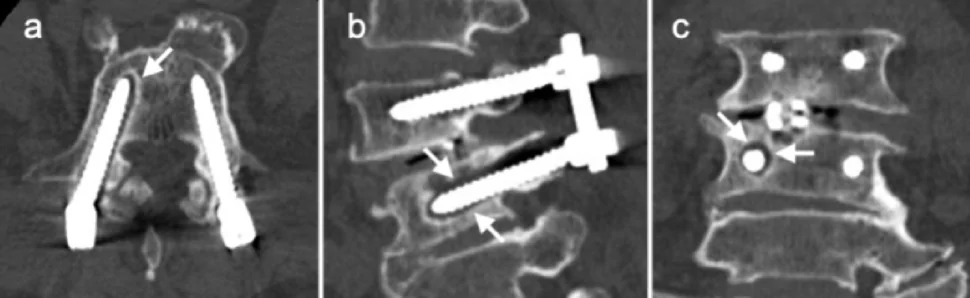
Assessment of pedicle screw (PS) loosening. (a) Axial, (b) sagittal, and (c) coronal CT images. PS loosening was defined as the presence of a radiolucent zone (arrows) measuring ≥ 1 mm in width surrounding the screw, observed in at least one CT plane.
